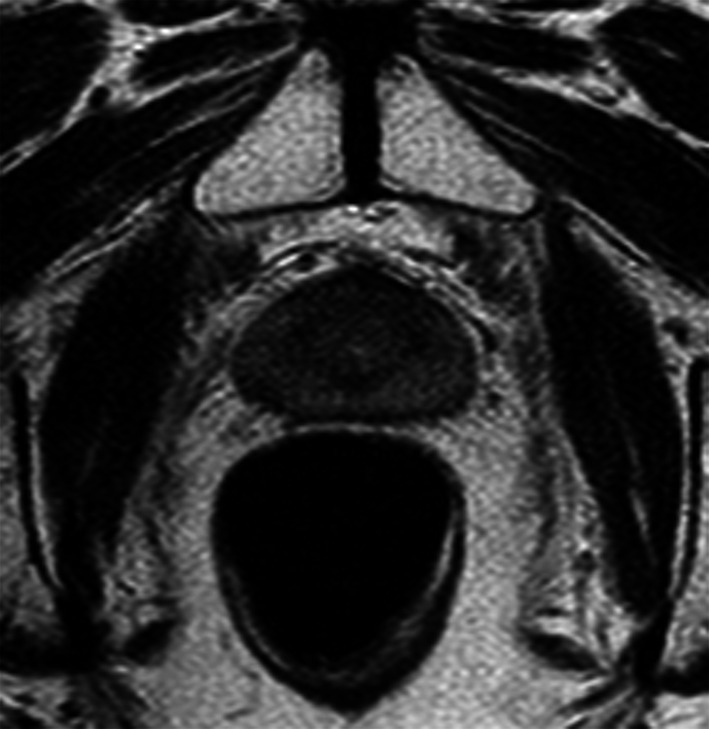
Follow-up MRI scan performed by a 67-year-old man with low-risk prostate cancer 12 months after external beam RT. Axial T2-weighted image shows the loss of zonal differentiation of the prostate
